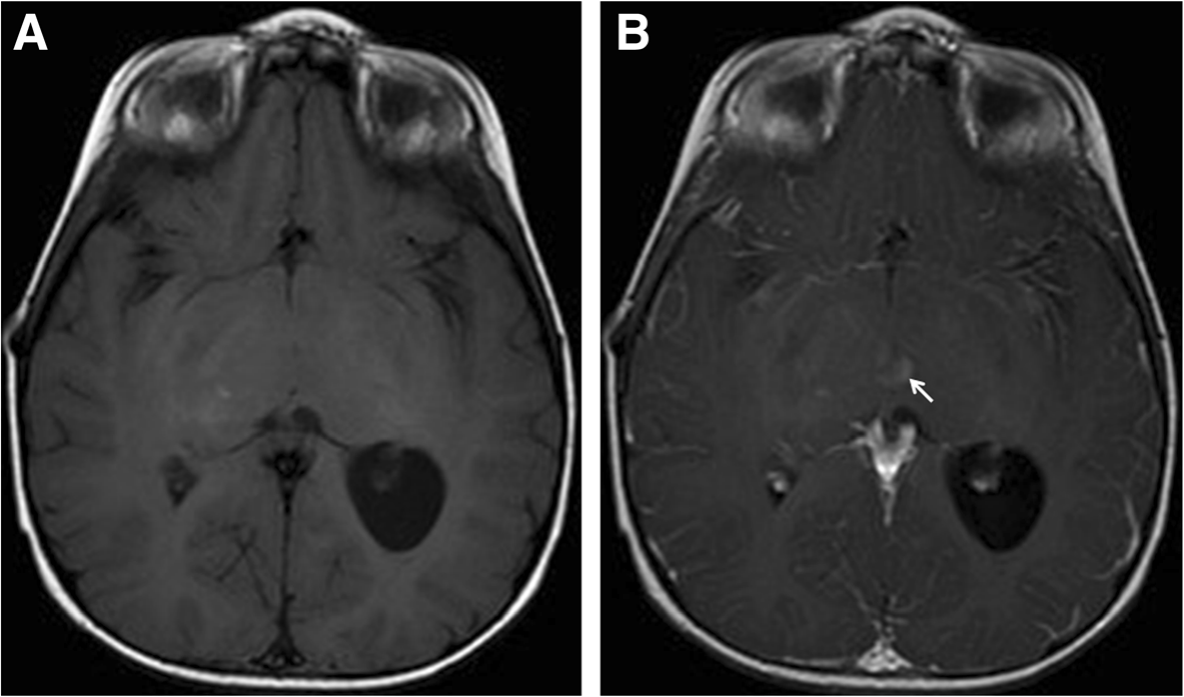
An axial T1-weighted MRI in a two-year old male with NF1 without contrast (A) and with contrast (B) showing an enhancing lesion within the hypothalamus (arrow)
